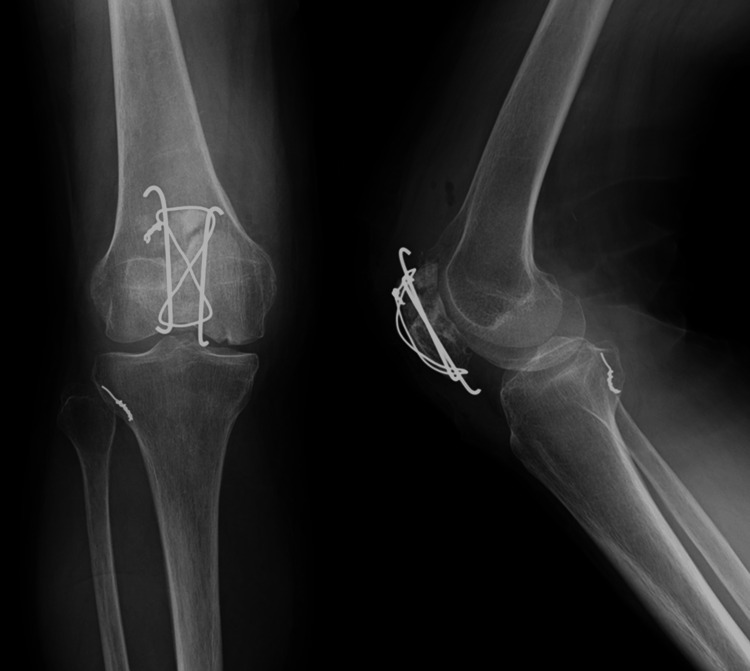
Radiographs (AP and lateral views) after wire retrieval AP, anteroposterior.
